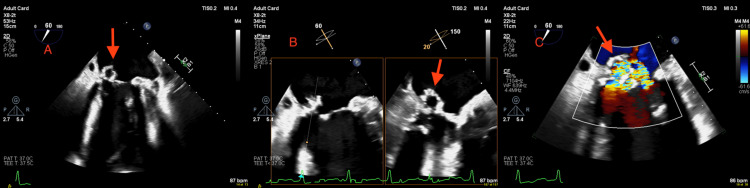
Transesophageal echocardiogram (A) Mid-oesophageal two-chamber view showing formation of PMVL aneurysm; (B) X plane assessment of PMVL showing aneurysm formation; (C) Colour Doppler assessment of MV showing MR due to perforation of mitral valve aneurysm. PMVL: posterior mitral valve leaflet; MR: mitral regurgitation; MV: mitral valve
